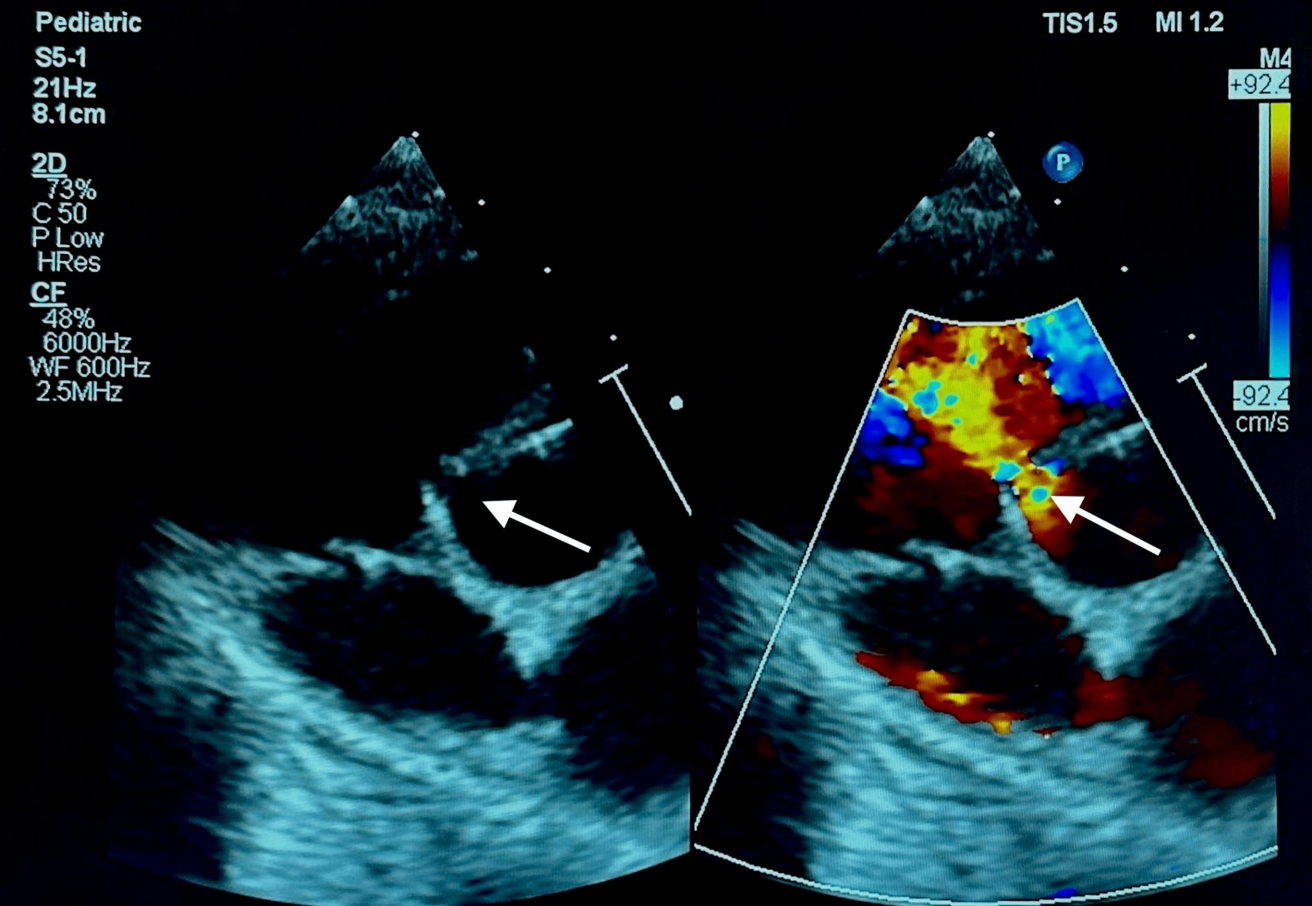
Echocardiogram revealing small, 2mm, perimembranous ventricular septal defect with mild mitral and tricuspid regurgitation and an intact atrial septum. Left ventricular systolic function was preserved with no evidence of hypertrophic obstructive cardiomyopathy.
